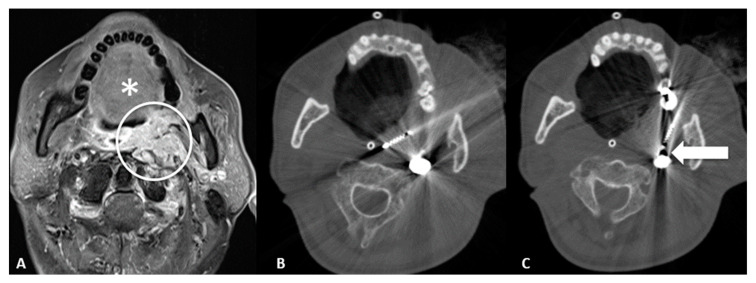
65-year-old male with trismus, gagging, airway compromise, and bleeding episodes due to recurrent nasopharyngeal carcinoma of the left retropharyngeal/paravertebral space with circumferential carotid artery involvement, after previous radiation therapy and left neck dissection. (A) T1-weighted postcontrast axial MRI scan showing malignant involvement of the left retropharyngeal space (circle). Notice the tongue (*) which can flop backward during the general anesthesia and could contact the ablation site; care must be taken in blocking the tongue prior to ablation. (B) Axial CT scan showing cryoablation needle placement through the retromolar trigone. (C) Axial CT scan showing the cryoablation needle near the previously embolized left carotid artery (arrow), which can lead to diving reflex.
